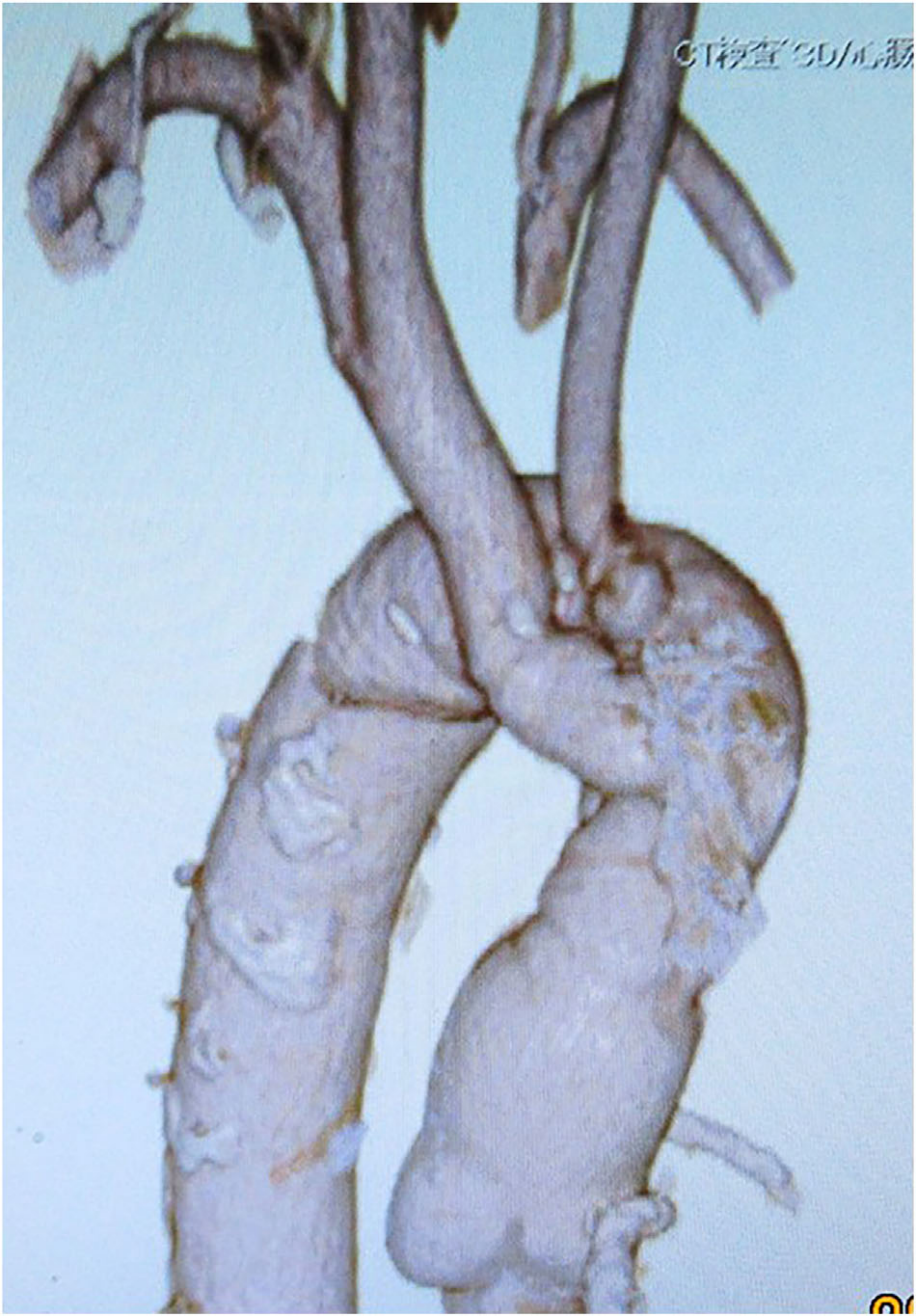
Postoperative 3‐dimensional computed tomography image acquired 36 months postoperatively. There are no stenoses, no calcifications, no thrombus formation, and no pseudoaneurysms of the neo‐aorta or its branches
